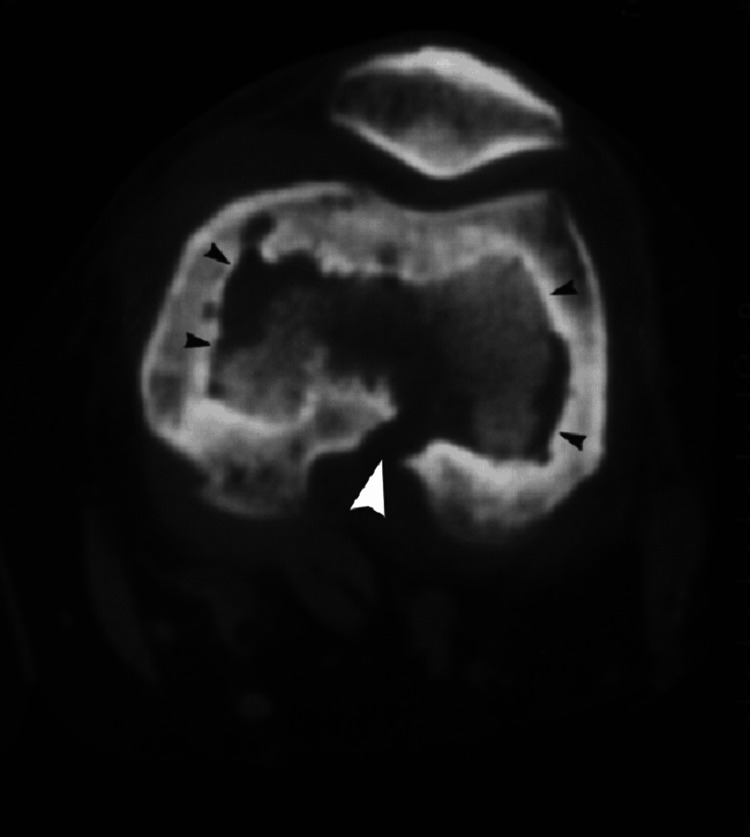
Transaxial CT image shows osteomyelitis of tibia with sequestrum formation.
